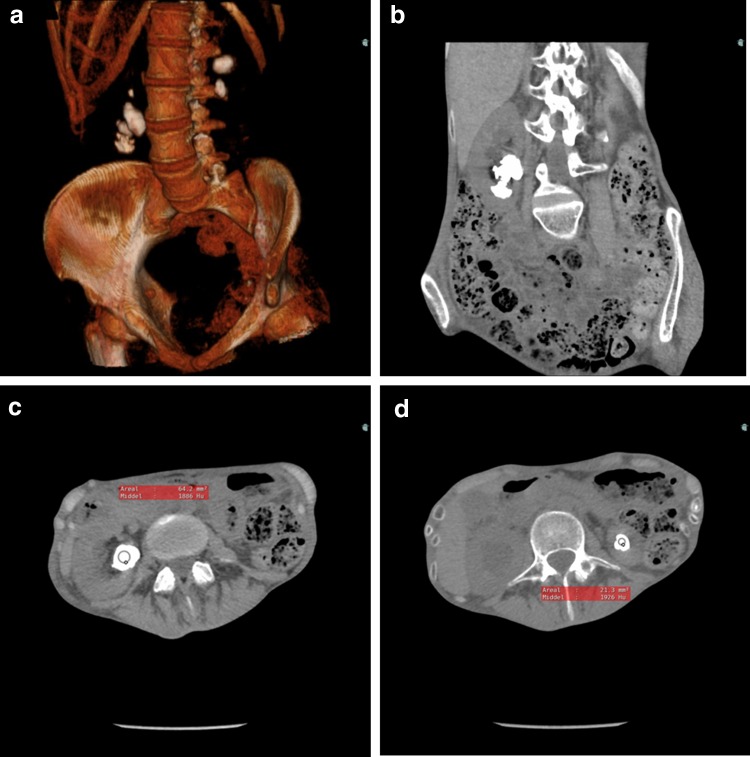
Three-dimensional reconstruction (a), coronal (b), and axial (c, d) CT images of bilateral calcite-nephrolithiasis in a malnourished anorectic woman. The stones were homogeneous with Hounsfield units around 1900.
